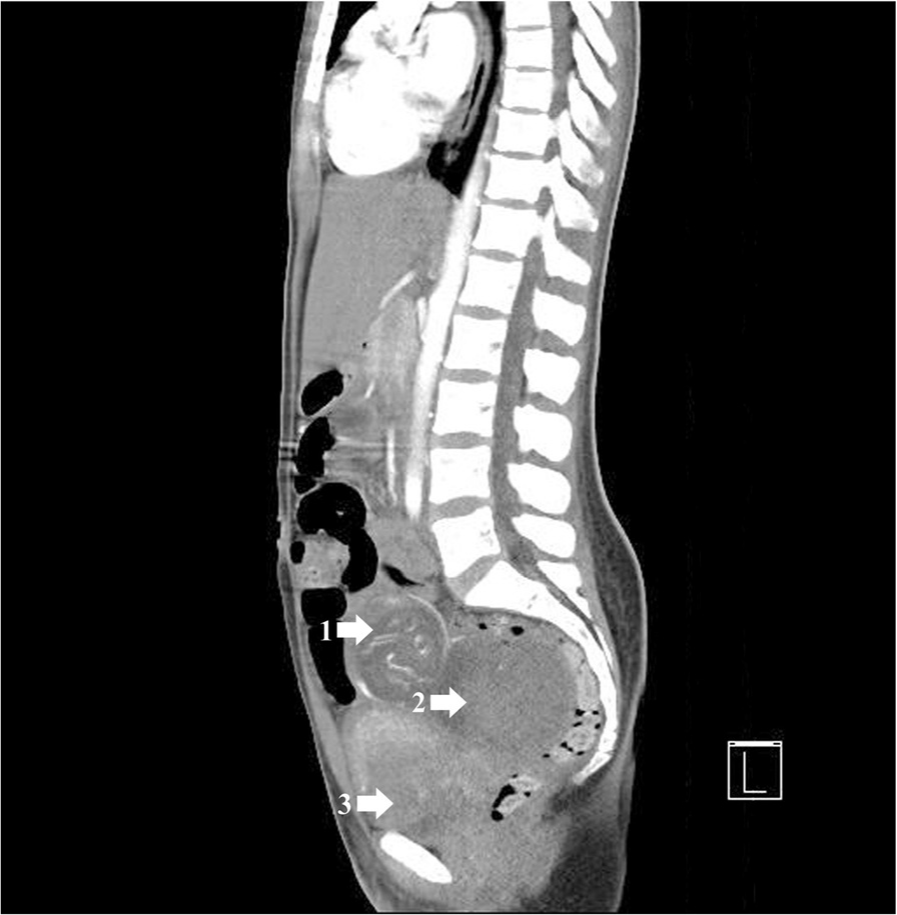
Computed tomography angiogram of abdomen and pelvis. 1 Intra-abdominal pregnancy. 2 Hematoma in cul-de-sac. 3 Uterus
